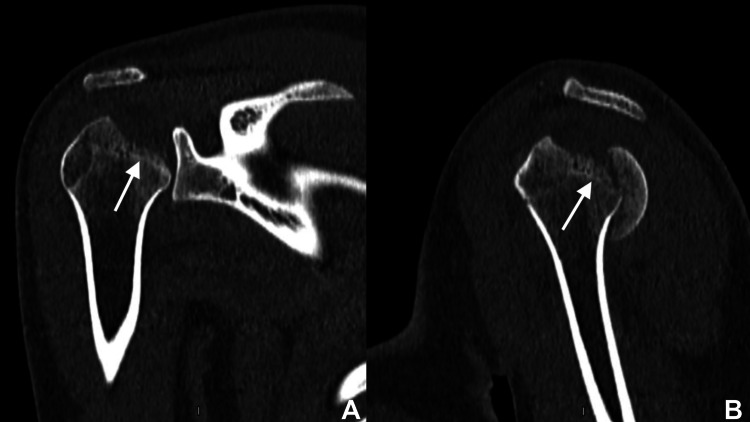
Initial CT showing the coronal (A) and sagittal (B) slides The white arrows signal a fracture line through the physeal scar of the proximal humerus.
